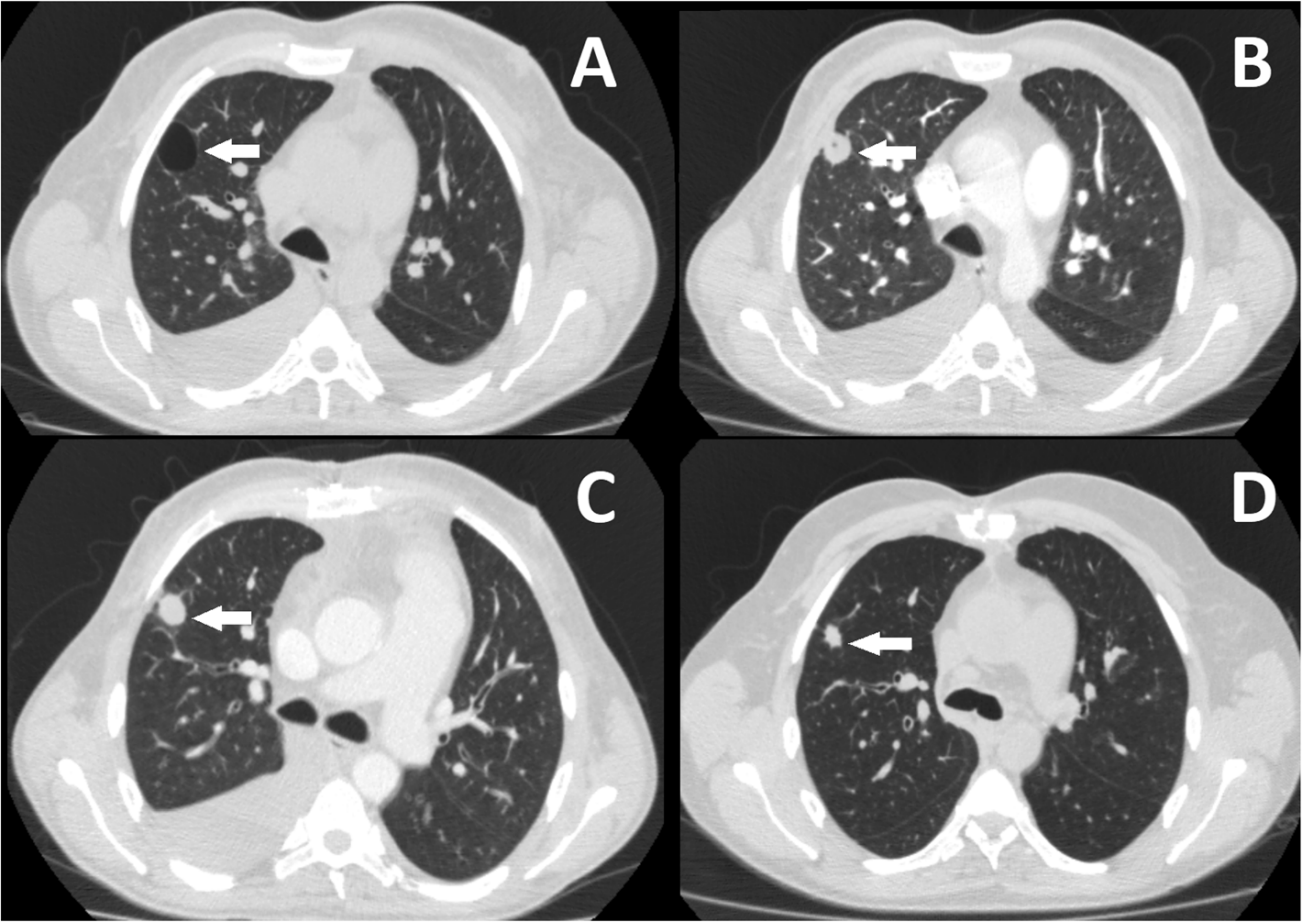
Evolution of pulmonary lesions over time by high resolution computed tomography. a – 20-01-2014; b – 6-11-2015; c – 29-12-2015; d – 15-04-2016. The white arrow indicates localization of pulmonary abscess
